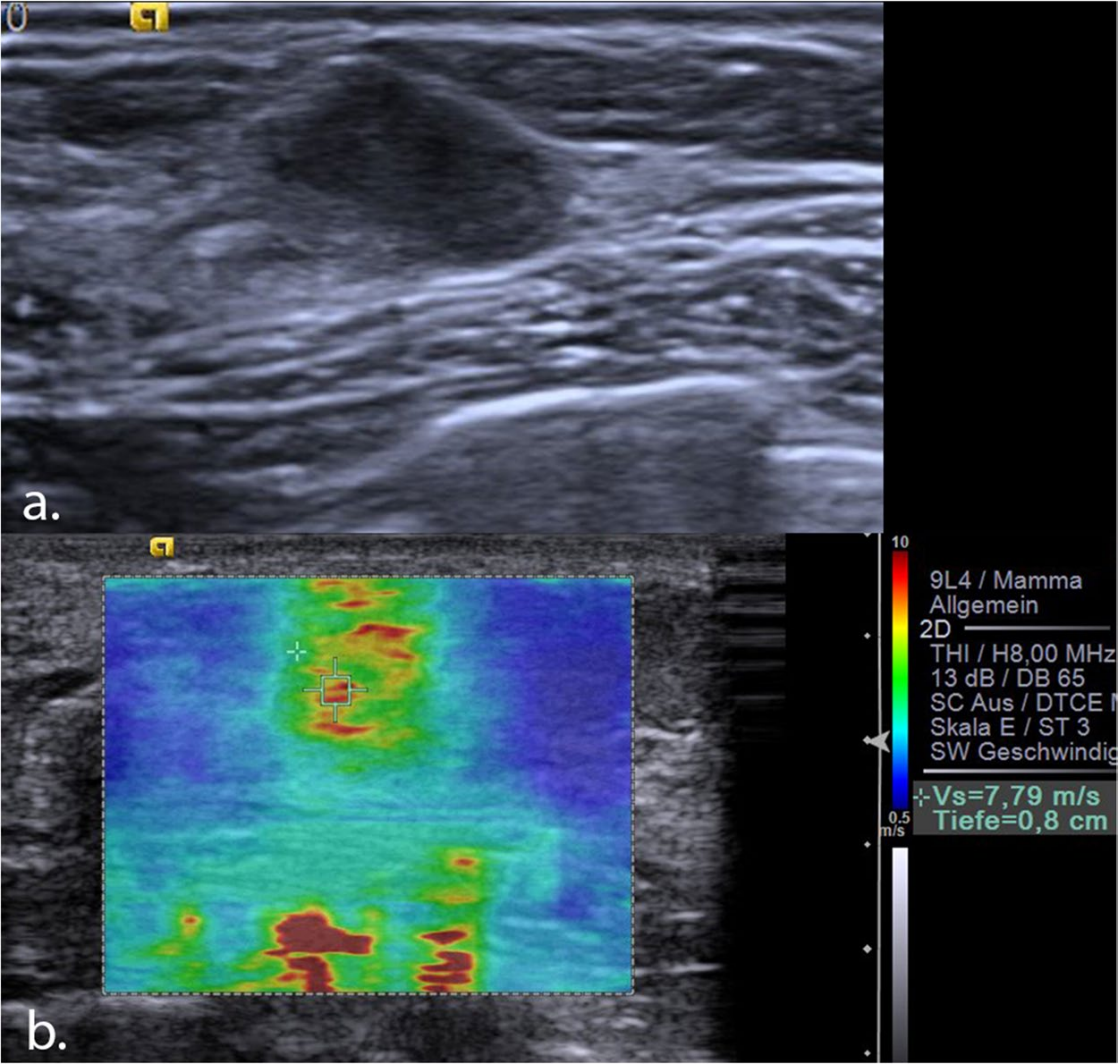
(a) Invasive ductal carcinoma Grade 1 seen in B-mode ultrasound as an 8 mm, hypoechoic, circumscribed lesion, which was initially classified as BI-RADS 3. (b) VTIQ elastography shows a very hard lesion (scale adjusted to 10 m/s) with a maximum shear wave velocity of 7.79 m/s (above the “rule-in” threshold), indicating a BI-RADS 5 tumour. VTIQ: Virtual Touch IQ, ROI: region of interest.
